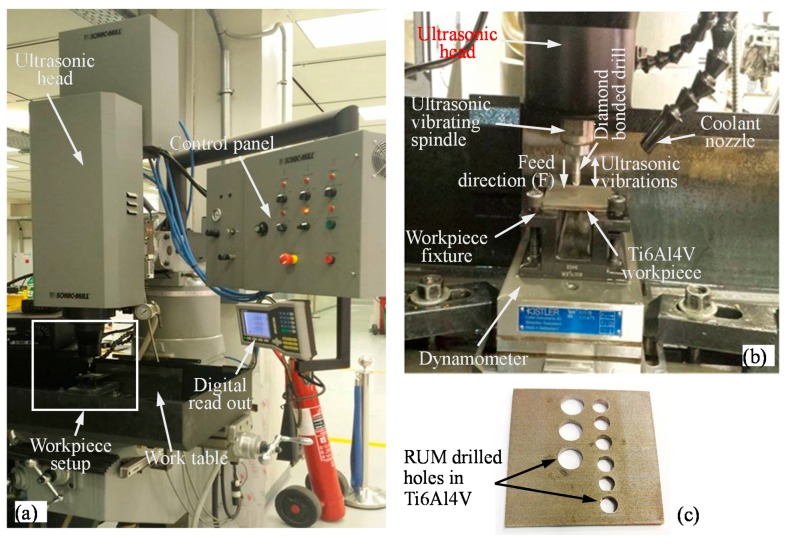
(a) Rotary ultrasonic machine used for experiments; (b) Rotary ultrasonic machining setup; (c) A Ti6Al4V work piece with drilled holes.
